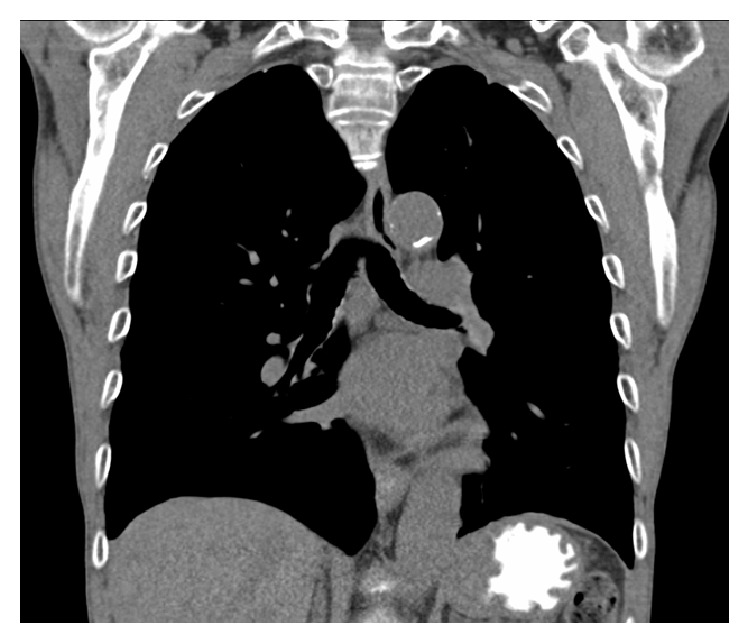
CT scan of the chest without intravenous contrast (coronal view) showing complete resolution of the right sided pleural effusion.
